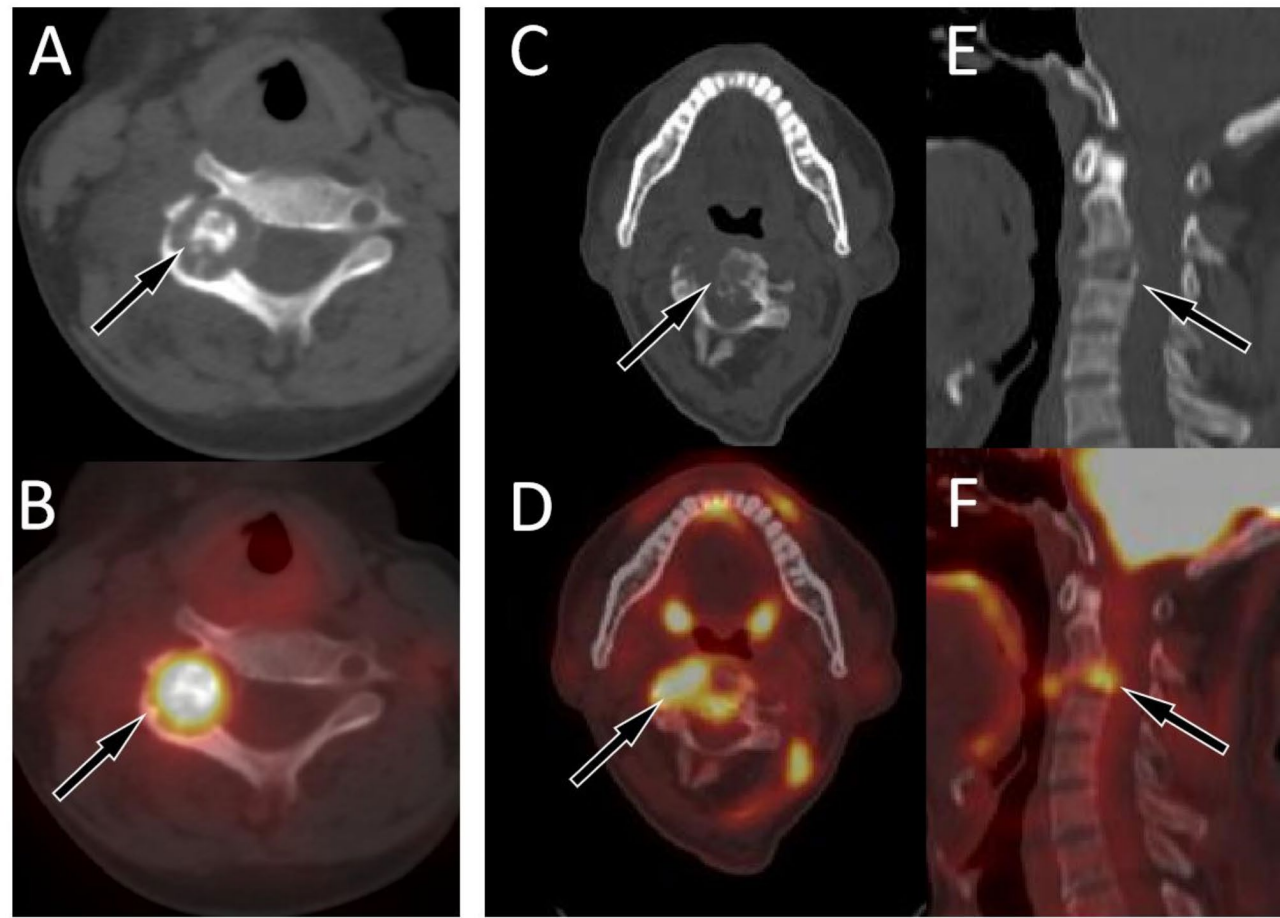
(A,B) A 10-year-old girl with typical PET/CT findings of osteoblastoma; (A) Axial CT revealed osteolytic bone destruction in the right vertebral plate of the fifth cervical vertebra, with nodular ossification at the center of the lesion (arrow); (B) The PET/CT fusion image of the corresponding area shows increased ^18^F-FDG uptake (arrow), with a SUVmax of 8.1. (C–F) A 18-year-old man with atypical PET/CT findings of osteoblastoma; (C) Axial CT revealed osteolytic bone destruction in the fourth cervical vertebra, with the main lesion located in the vertebral body and multiple residual bone ridges visible within the lesion (arrow); (D) The PET/CT fusion image of the corresponding area shows increased ^18^F-FDG uptake (arrow), with a SUVmax of 6.8; Sagittal (E CT; F PET/CT) reveals compressive changes of the vertebral body (arrows).
